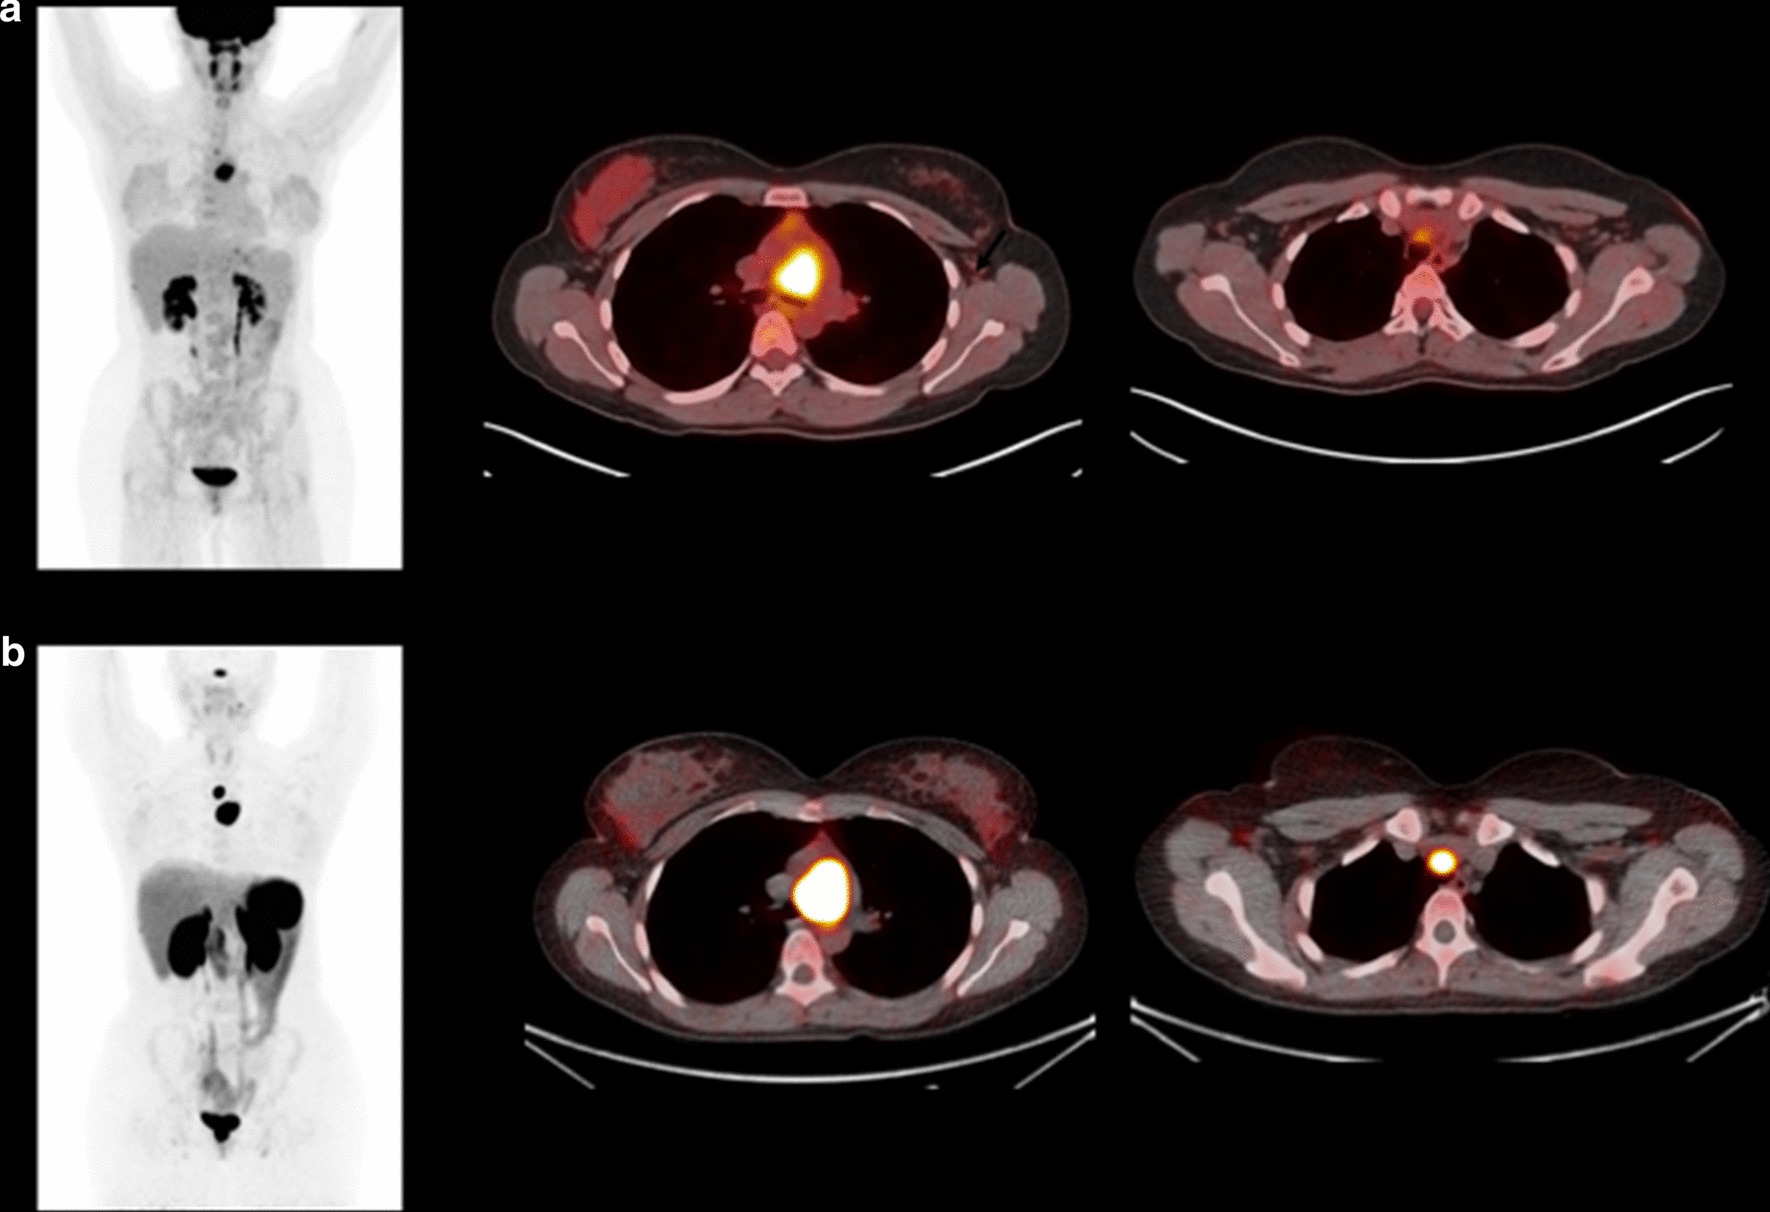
Case 012. Panel A, [^18^F]FDG PET/CT MIP image and fused transaxial views showing a highly avid mediastinal mass and low-level uptake of an adjacent lymph node (C). This imaging was performed as part of surveillance for disease recurrence given a background of a resected wild-type GIST, and the mediastinal mass was a presumed recurrence of this earlier tumour. Panel B, ^68^ Ga-DOTATATE PET/CT, same views as above. Very intense uptake in the mediastinal mass (SUVmax 45) and adjacent lymph node (SUVmax 40). This patient had normal plasma metanephrine and 3-methoxytyramine values on biochemical testing. Biopsy confirmed a non-secreting mediastinal dSDH paraganglioma case 012
